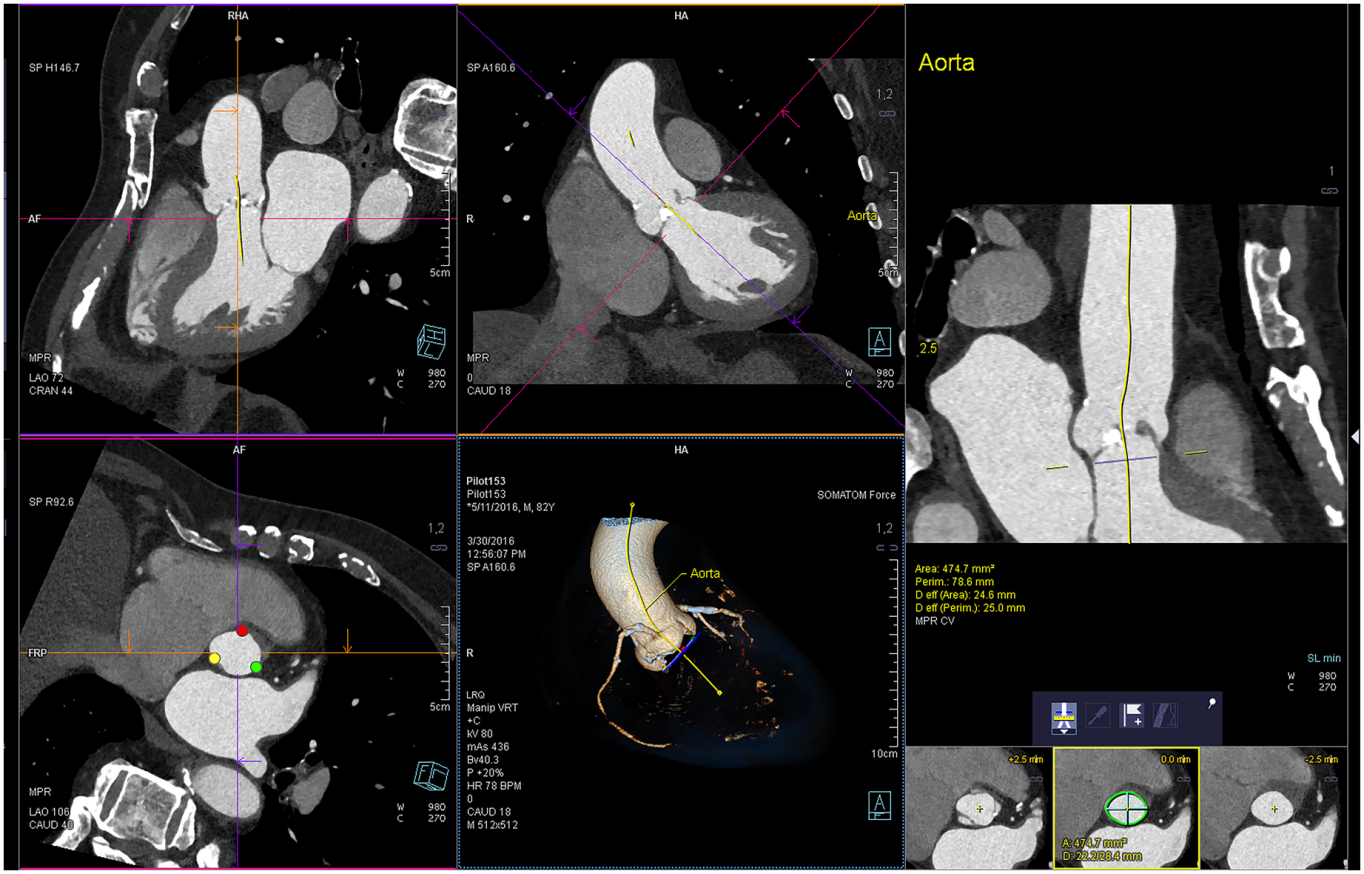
Automatic aortic annulus plane of prototype version of syngo.CT Cardiac Planning. Syngo.via^™^ VB20A, Siemens Healthcare GmbH, Forchheim Germany.
